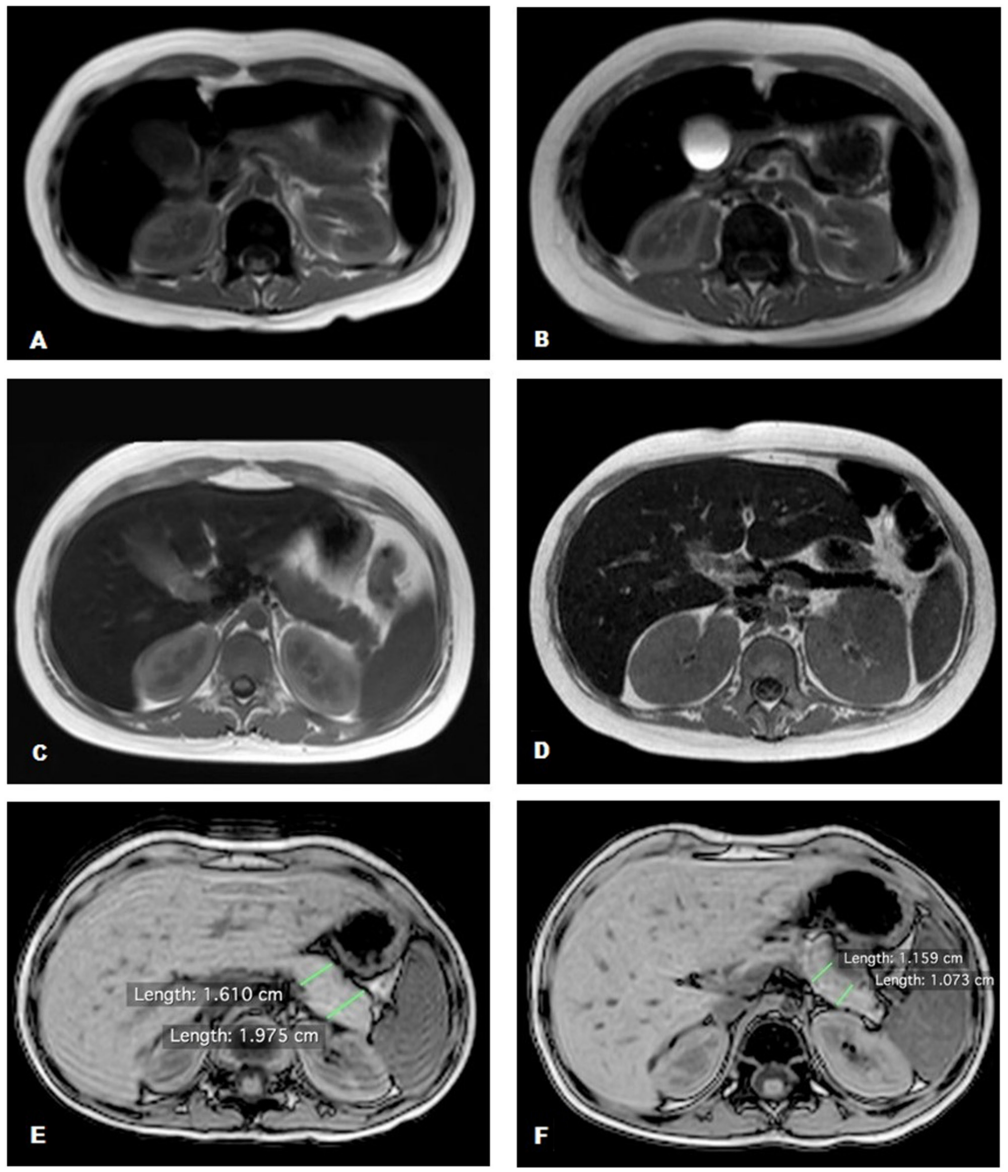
Abdominal MRI T2 fast field echo sequences (A, C, E) Normal pancreas signal and volume; (B, D) hypointense pancreas signal with reduction of pancreatic volume; (F) normal pancreas signal with a reduction of pancreatic volume.
